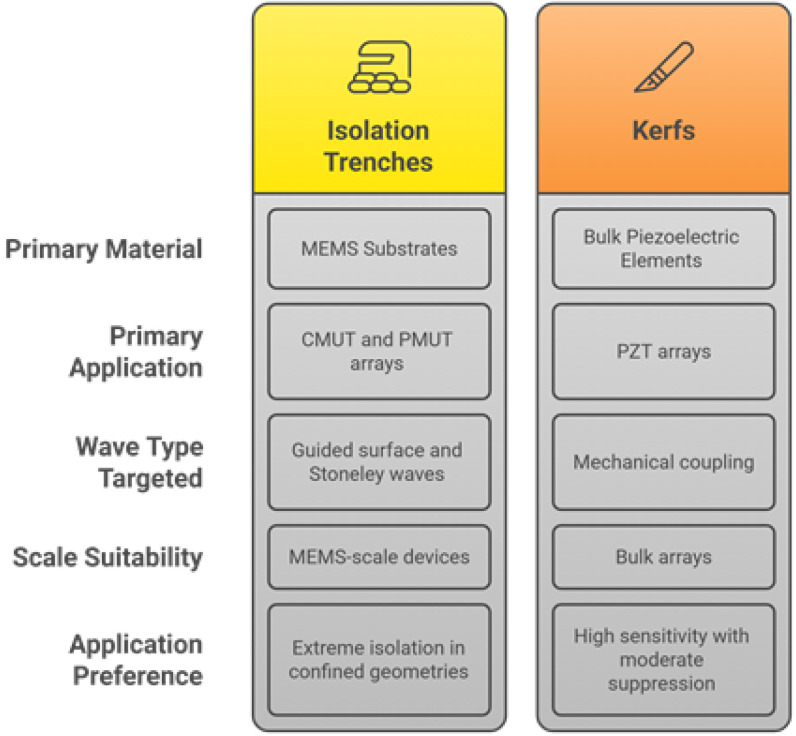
Comparative performance of isolation trenches and kerfs in reducing acoustic and mechanical crosstalk in ultrasonic transducer arrays.
